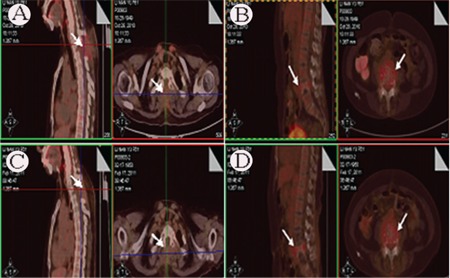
PET-CT of the patient revealed FDG uptake at second thoracic (Figure 3A, C) and fourth lumbar vertabrae (Figure 3B, D).
